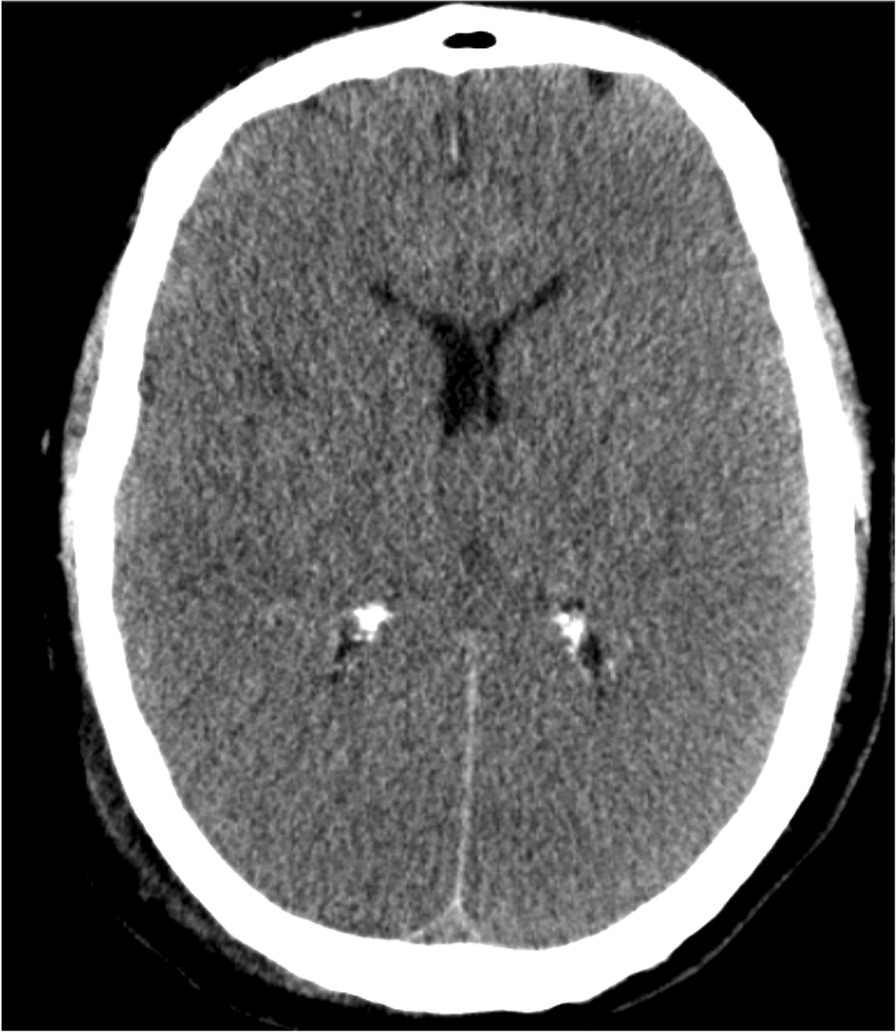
Computed tomography scan performed 4 days after the first scan shows diffuse cortical hypodensity of both cerebral hemispheres, with a more evident loss of grey-white matter differentiation with gyral effacement and compression of lateral ventricles
